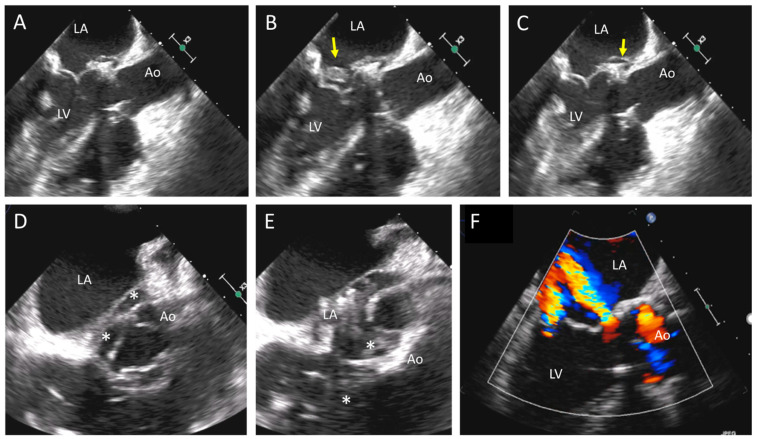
Transesophageal echocardiography of complex endocarditis in a patient with biological mitral and aortic prosthesis. (A,B) From a mid-esophageal view, there is a normal leaflet appearance (A), and in a systolic frame, there is the presence of vegetation (B, arrow). (C) The arrow indicates an aortic pseudoaneurysm in the mitral–aortic intervalvular region. (D,E) Short axes of the aortic valve (upper-esophageal view) showing a large abscess of the periaortic tissue (asterisks). (F) Color-Doppler also shows a LVOT/left atrial fistula located at the base of the mitral valve. Ao, aorta; LA, left atrium; LV, left ventricle; LVOT, left ventricle outflow tract.
